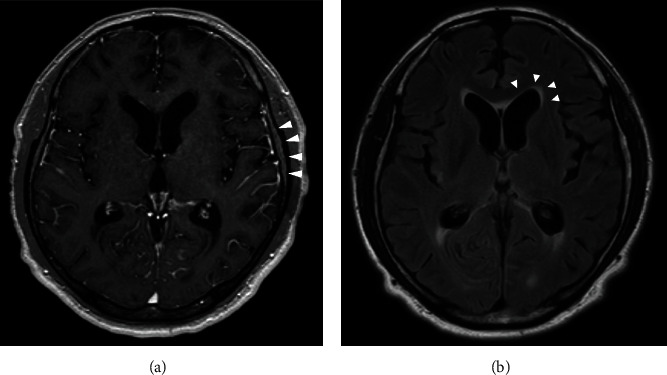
Dynamic contrast-enhanced MRI showing LMC of the cerebrum, mesencephalon, and cerebellum (a). Infiltrating tumor cells can be identified along the cerebral cortex on T2 fluid-attenuated inversion-recovery imaging (b) (arrows).
